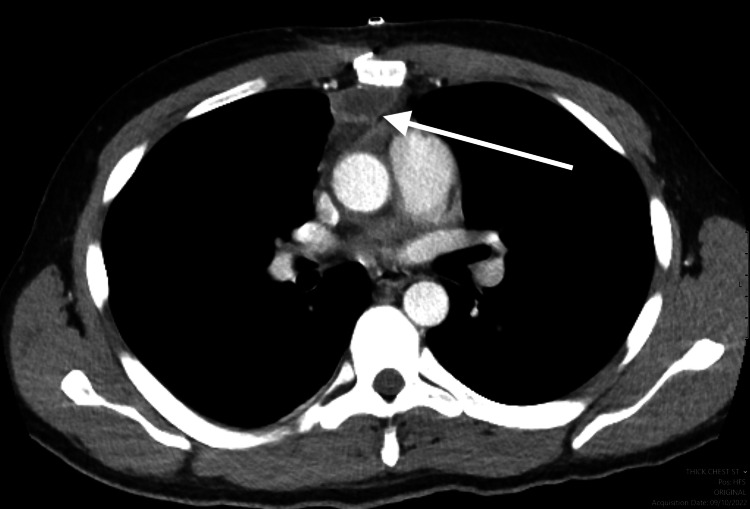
Axial CT chest reveals fluid collection with peripheral rim enhancement in the retrosternal region. Interval removal of bullet from the right ventricle postoperatively.
